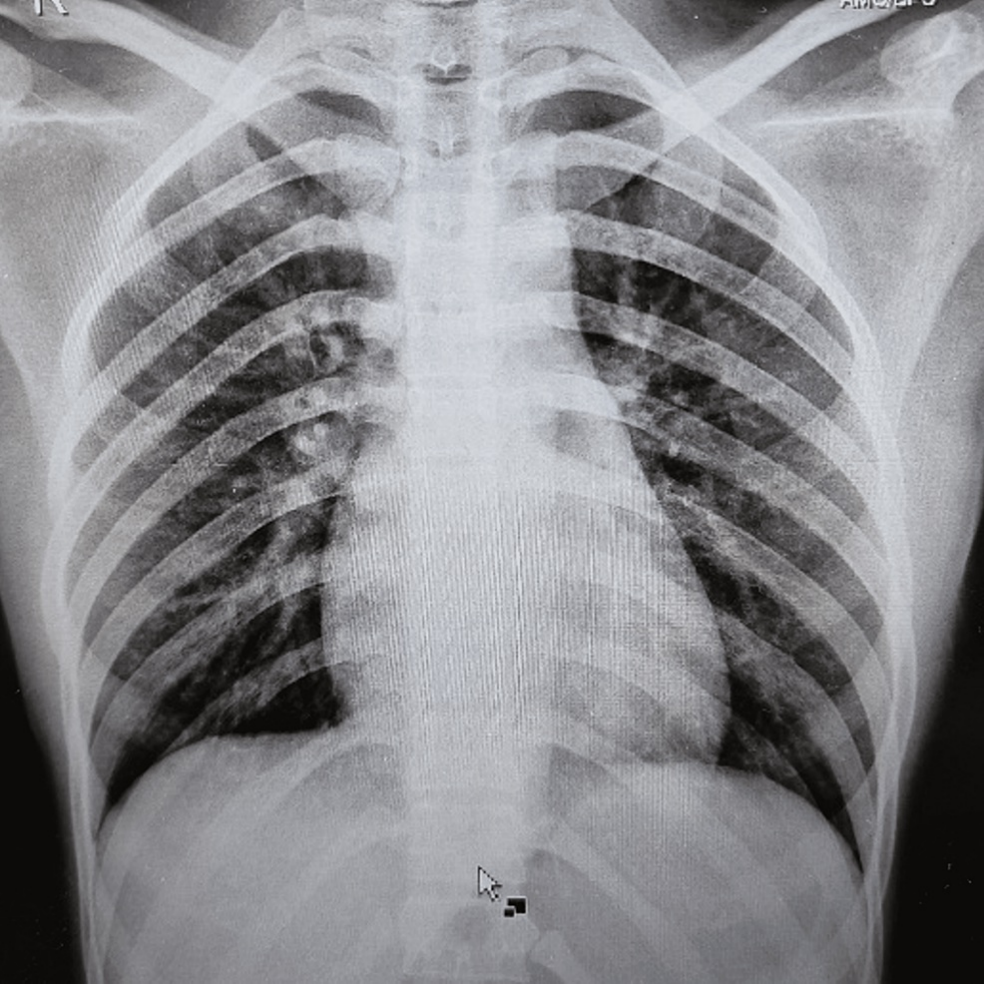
Chest radiograph - PA view showing normal lung fields except for a few calcified lymph nodes PA: posteroanterior
